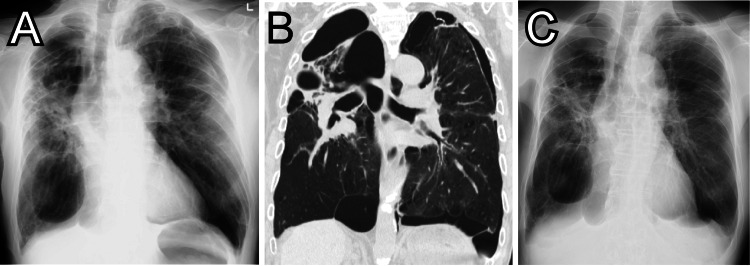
Chest X-ray and computed tomography. X-ray (A) and computed tomography (B) of the chest on arrival showing a left-sided mild pneumothorax and pre-existent multiple giant bullae. (C)  Chest X-ray after 10 days showing improvement of the left-sided pneumothorax.
